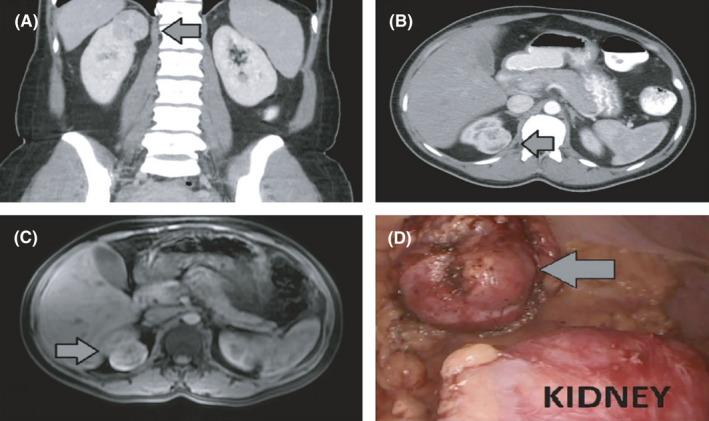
(A‐C) Abdominal computed tomography and magnetic resonance imaging show a large exophytic heterogeneous mass in the upper pole of the right kidney. (D) Intraoperative image of the renal mass
